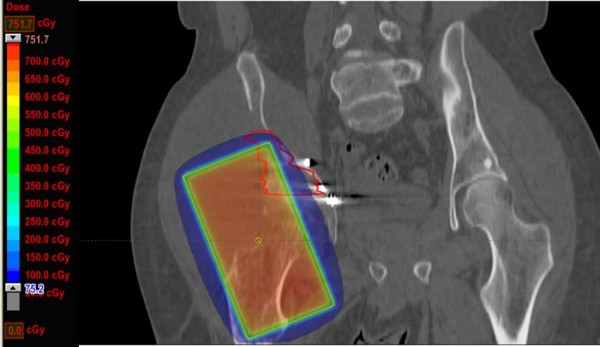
The original 8 × 15 field was digitally reconstructed and superimposed on a CT scan following the patient’s diagnosis of osteosarcoma, and the treatment plan was subsequently recreated. The region of greatest bony destruction was thought to be the epicenter of the tumor, and was specially contoured on the diagnostic CT scan. The volume appears to be located at the edge of the radiotherapy field, including both areas that received >700 cGy and <100 cGy.
